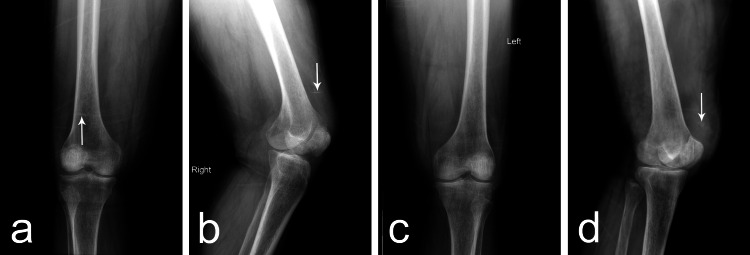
Preoperative radiographs of both knees. (a) Anteroposterior and (b) lateral views of the right knee. (c) Anteroposterior and (d) lateral views of the left knee. Moderate bilateral degenerative changes consistent with Kellgren-Lawrence grade 2 osteoarthritis are present. Small avulsion fragments adjacent to the superior poles of the patellae (arrows), together with bilateral patella baja, are visible and represent subtle but important radiographic signs of quadriceps tendon rupture.
